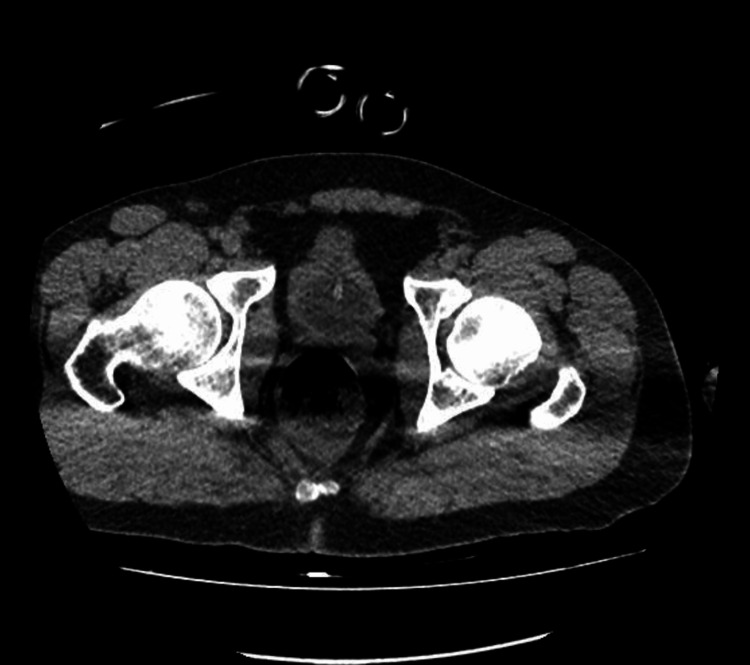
Pelvic CT scan showing no abnormalities. CT scan of the upper abdomen and pelvis was performed using a multidetector helical technique. Images were obtained before and after intravenous administration of the contrast medium.
